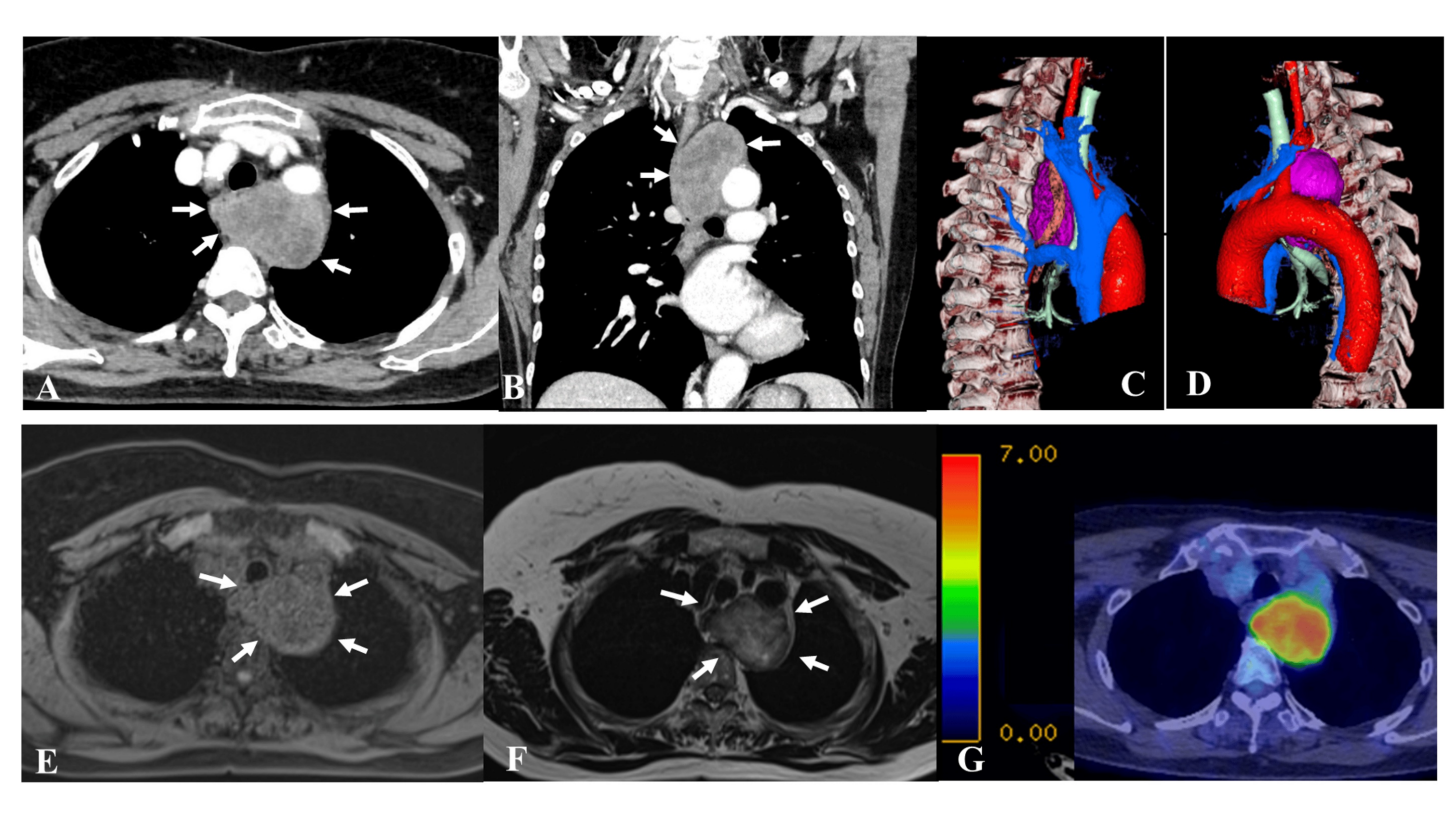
Radiological examination Chest-enhanced CT (A, B) and 3D-CT (C, D) showed a mass (diameter: 7.4 cm) located close to the posterior of the trachea with a clear border and heterogeneous contrast effect. The tumor was in contact with the medial side of the aorta, the dorsal side of the left subclavian artery, and the azygos venous arch, and severely compressed the esophagus to the right. Chest contrast MRI showed that the mass was not continuous with the esophagus and showed low T1 (E), heterogeneous high T2, and a low capsular signal on the periphery (F). On FDG-PET (G), the mass showed FDG accumulation with SUV_max_ of 8.2. 3D: Three-dimensional; CT: Computed tomography; FDG: 18F-fluorodeoxyglucose; MRI: Magnetic resonance imaging; PET: Positron emission tomography; SUV_max_: Maximum standard unit value.
